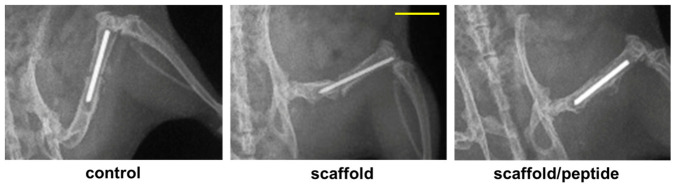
Representative radiographs 6 weeks postoperatively. Compact bone appears more radiopaque. Complete bone regeneration is observed only when the scaffold is coated with the PTN_48-56_ peptide. Control: incomplete bone healing when no scaffold was used; Scaffold: incomplete bone healing when an uncoated scaffold was used; Scaffold/peptide: successful bone healing when a scaffold coated with the PTN_48-56_ peptide was used. All photos have the same magnification, and the yellow scale bar corresponds to 1 cm.
